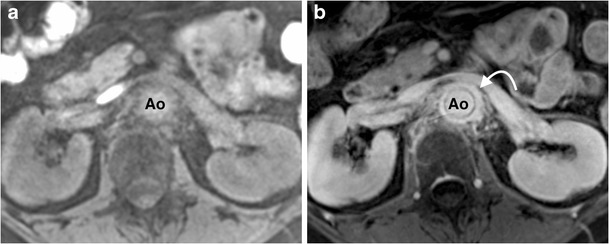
Selected axial images from T1-weighted 3D spoiled gradient echo sequence with a fat selective prepulse sequence pre- (a) and post- (b) gadolinium in a 55-year-old woman with giant cell arteritis demonstrates enhancing circumferential mural aortic soft thickening (*arrow*) of the juxtarenal aorta (*Ao*) consistent with a large vessel vasculitis
